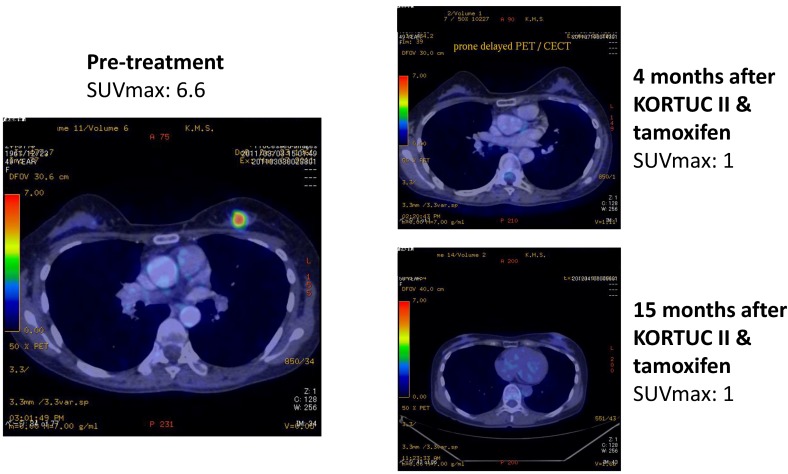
Case 40. 49 years old female patient with left breast cancer (cT2N0M0, ER: +, PgR: +, Ki-67 index: 24%) treated with radiosensitization (KORTUC II) and without systemic chemotherapy. Left: PET-CT image at pre-treatment. Tumor diameter is 24 mm and SUV-max value is 6.6; Right-upper: PET-CT image at 4 months following KORTUC II. Tumor is not recognized and SUV-max value on the region is approximately 1; Right-lower: PET-CT image at 15 months following KORTUC II. Tumor is not recognized and SUV-max value on the region is approximately 1.
